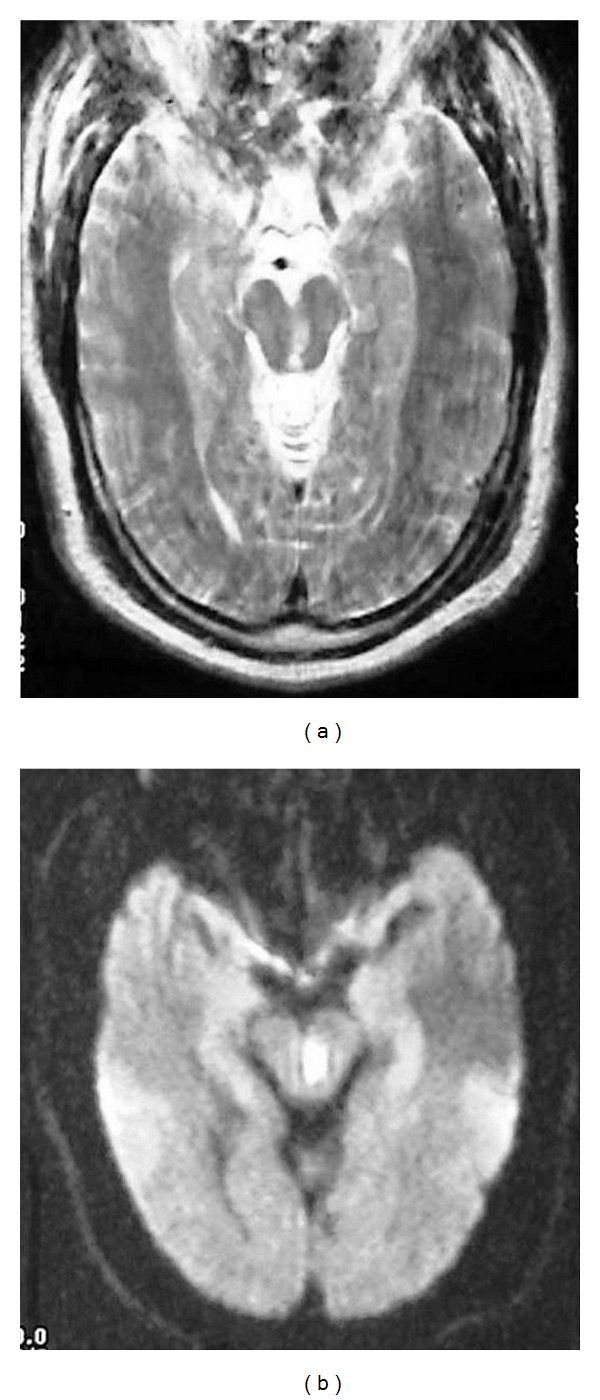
Claude Syndrome. Severely motion degraded axial T2-weighted (a) and DWI (b) images of the midbrain demonstrate a subacute infarct within the left midbrain tegmentum, with extension from the Sylvian aqueduct to the red nucleus. This patient presented with downward abduction of the left eye and right leg incoordination with poor gait. The visual disturbance is related to the involvement of the oculomotor nerve fascicles. Injury to the dentato-rubro fibers and/or red nucleus resulted in ataxia.
